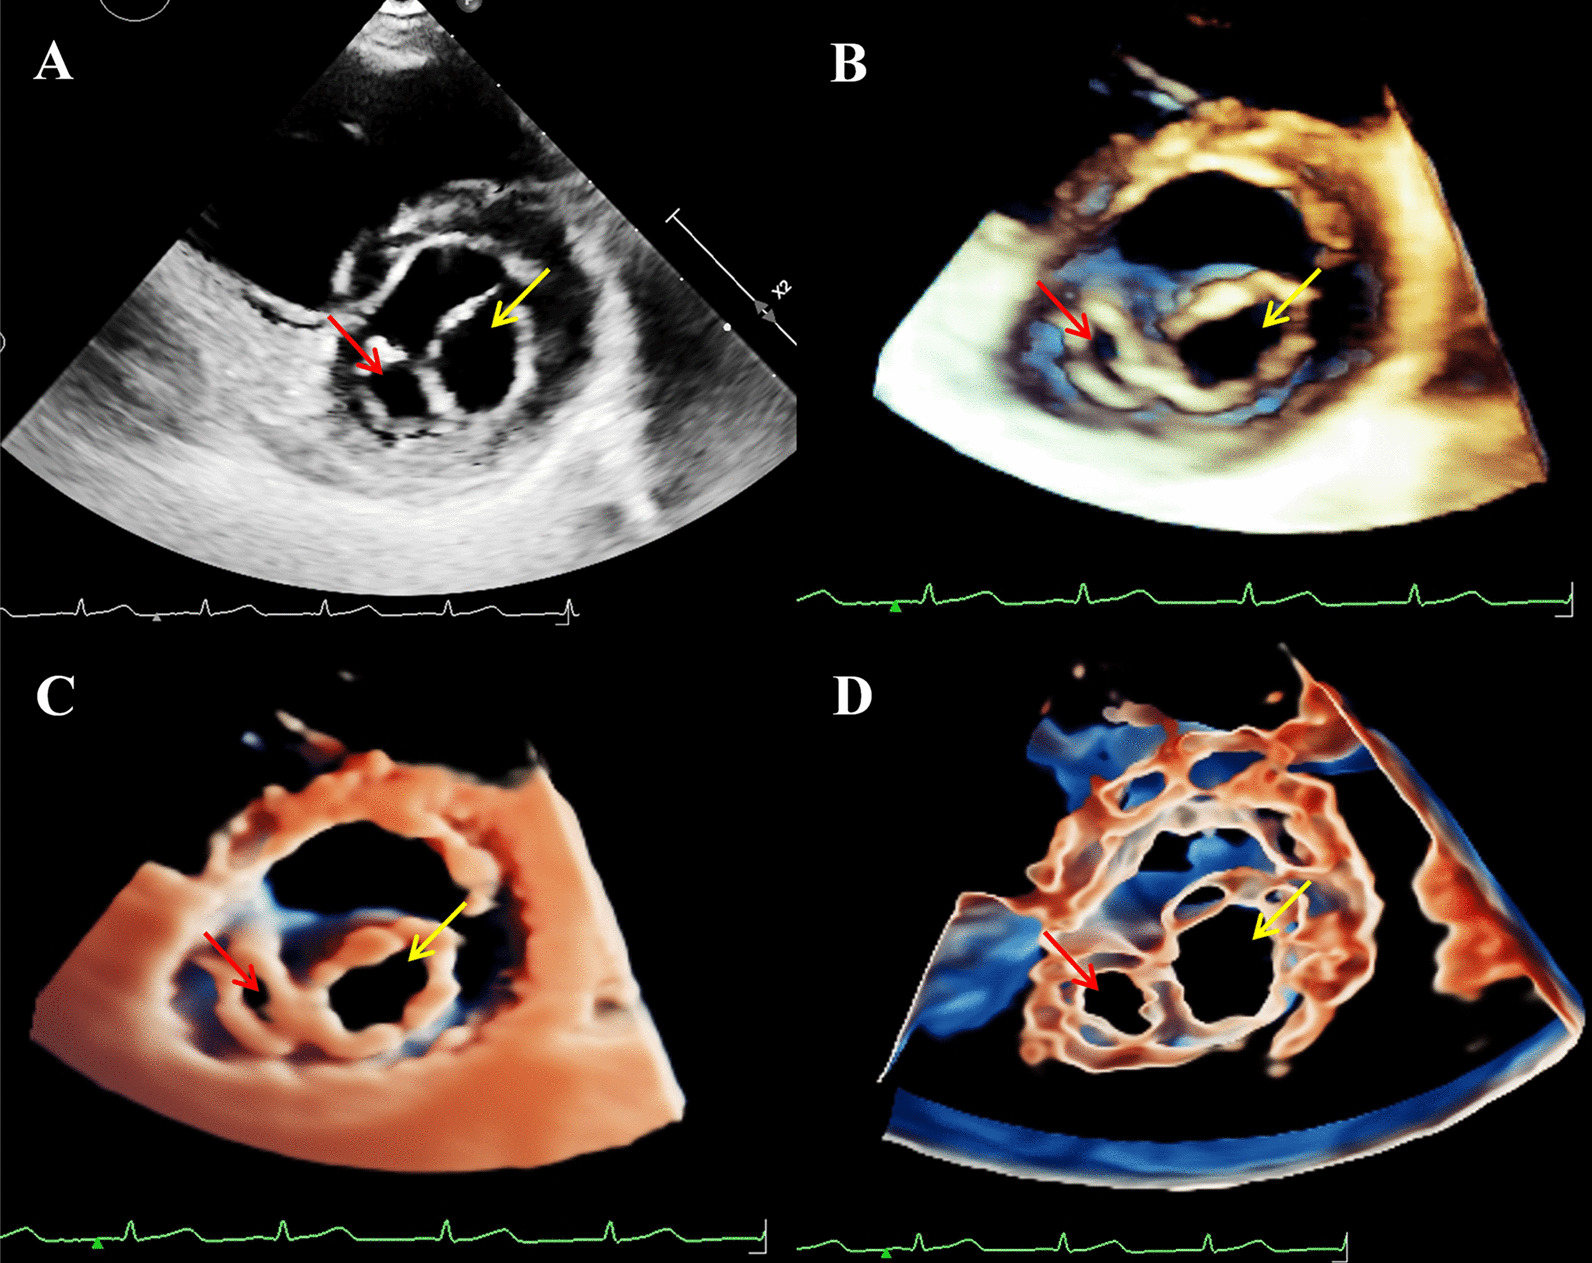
Double mitral orifices. A–D 2D-TTE, traditional 3DE, TrueVue, and TrueVue Glass images showing double mitral orifices viewed in left ventricular short axis section (mitral valve orifice level). Yellow arrows show orifice 1, red arrows show orifice 2. *2D-TTE* two-dimensional transthoracic echocardiogram, *3DE* three-dimensional echocardiography
